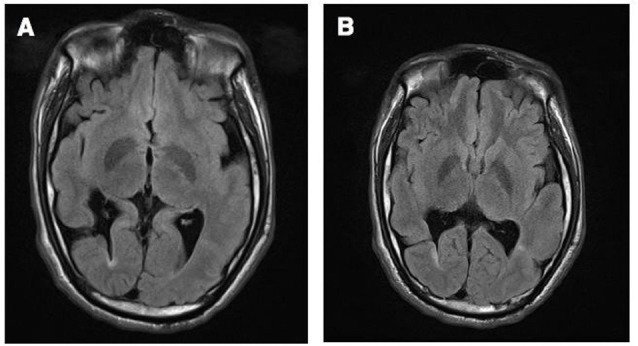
Brain magnetic resonance imaging (MRI) revealed a closed-lip schizencephalic cleft in the right hemisphere (A), and non-schizencephalic focal cortical dysplasia in the left hemisphere (B).
